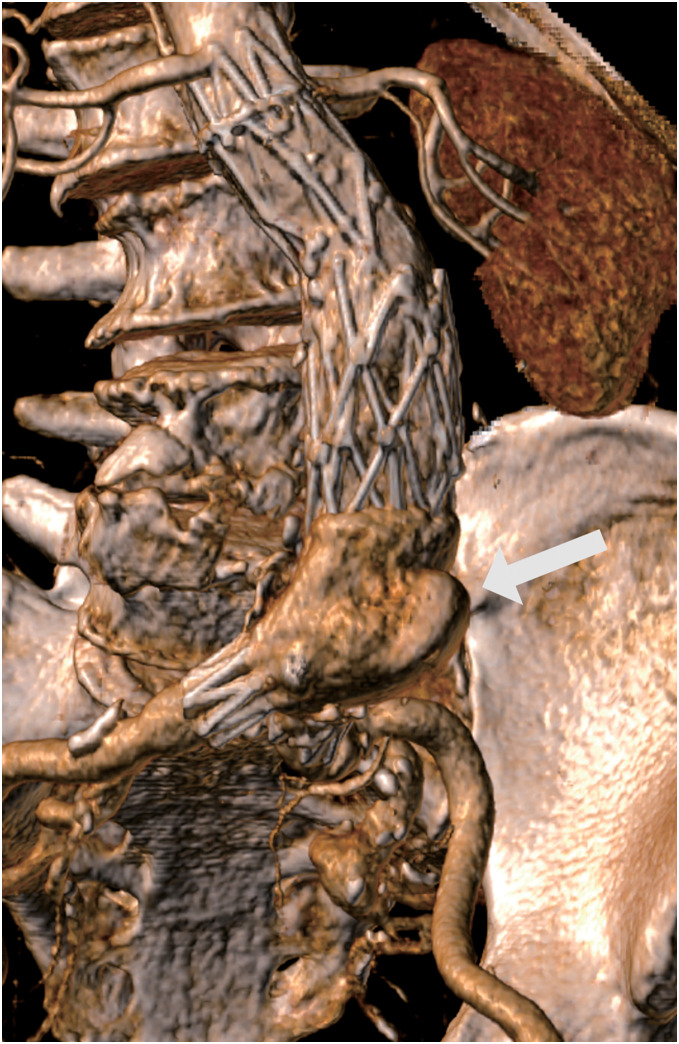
Fig. 1 Preoperative CT image. Preoperative contrast-enhanced computed tomography (CT) images of the abdominal aorta 3 years after AFX implantation. A type 3 endoleak was suspected (arrow).
